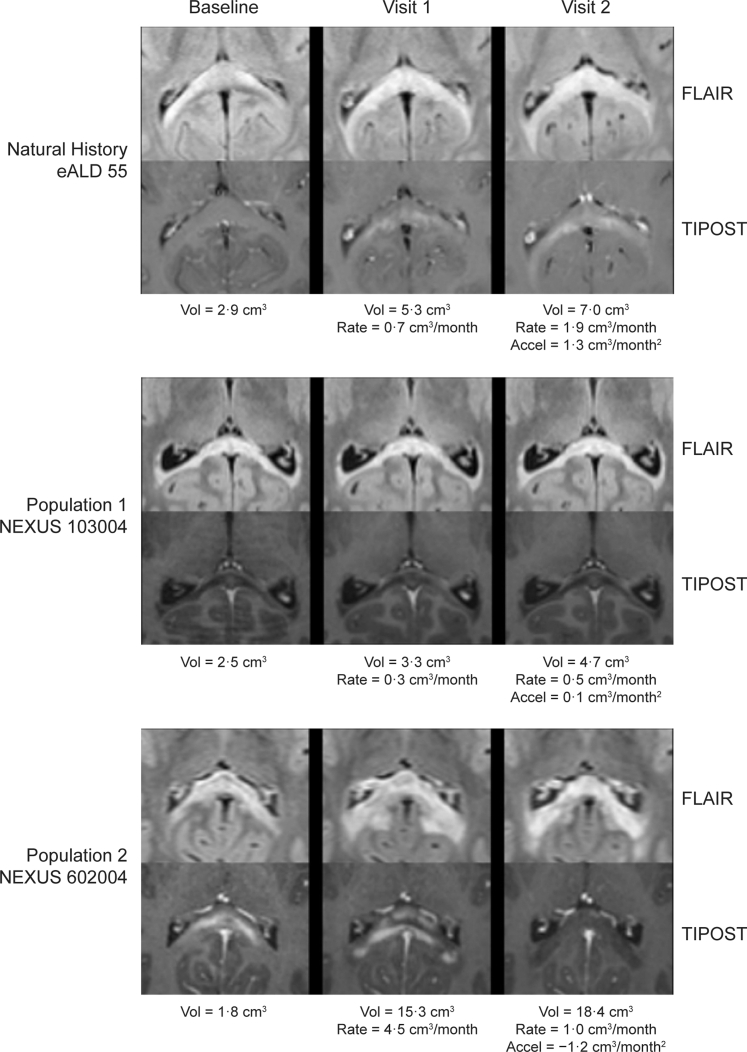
T2 FLAIR and T1-post contrast MRI scans from an untreated patient from a natural history cohort, and patients in populations 1 and 2 of the NEXUS study. Rows 1 and 2: early-stage lesion progression in an untreated 4-year-old patient at baseline, visit 1 (3·5 months) and visit 2 (4·4 months), demonstrating the development of lesional enhancement and lesion growth acceleration. Rows 3 and 4: lesion progression in an 8-year-old participant from Population 1 at baseline, visit 1 (3·0 months) and visit 2 (6·0 months), demonstrating a lack of lesional enhancement and slow lesion growth compared to natural history. Rows 5 and 6: lesion progression in a 6-year-old participant from Population 2 at baseline, visit 1 (3·0 months) and visit 2 (6·0 months), demonstrating resolving lesional enhancement and significant lesion growth deceleration inconsistent with the natural history of early-stage childhood cerebral adrenoleukodystrophy. Accel, acceleration; eALD, early-stage adrenoleukodystrophy; FLAIR, fluid-attenuated inversion recovery; MRI, magnetic resonance imaging; T1POST, T1-post contrast; Vol, volume.
